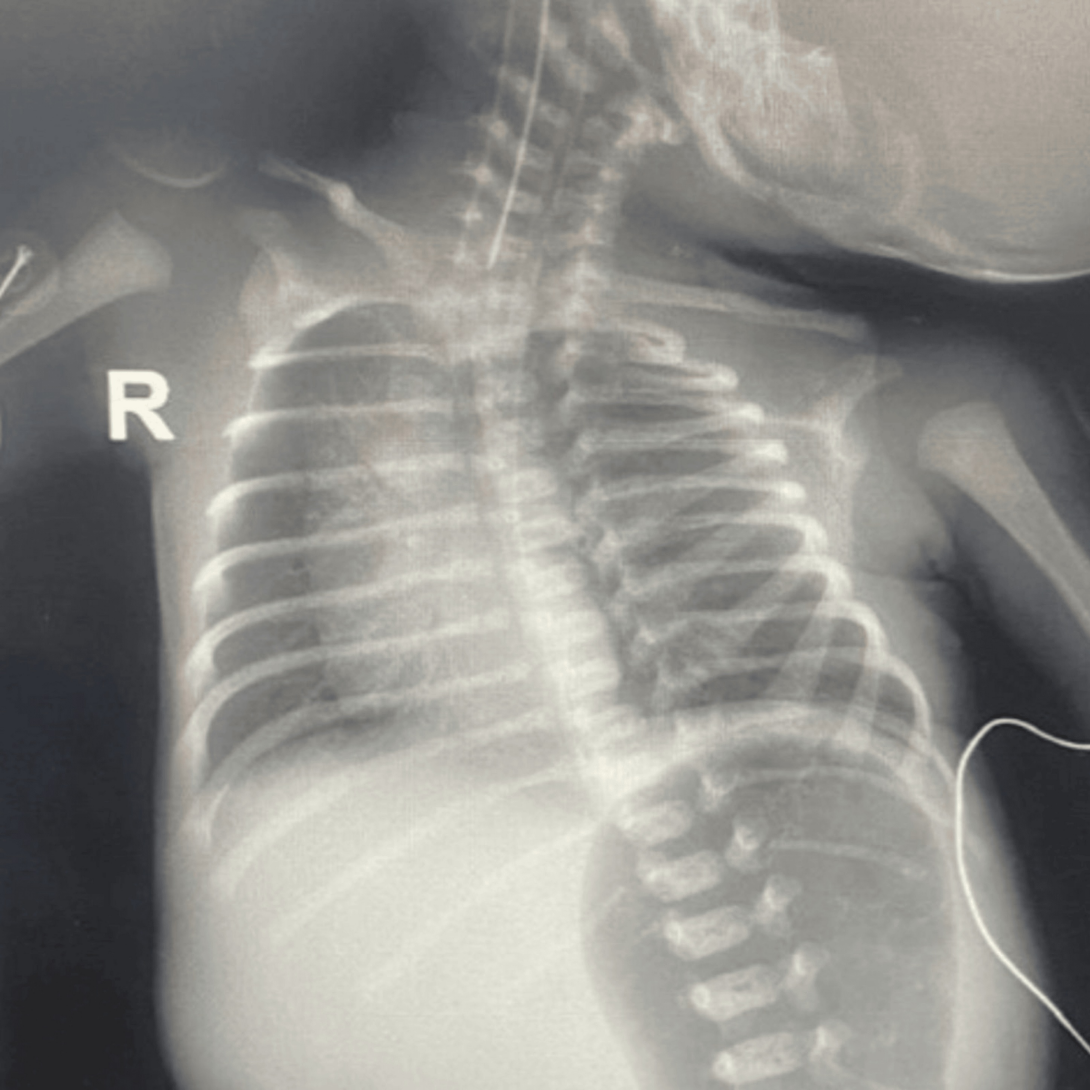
Preoperative abdominal X-ray demonstrating herniation of bowel loops through the umbilical defect. X-ray showing herniation of small and large bowel loops through a small umbilical abdominal wall defect. Air is seen in the dilated stomach. No solid organ herniation is evident.
